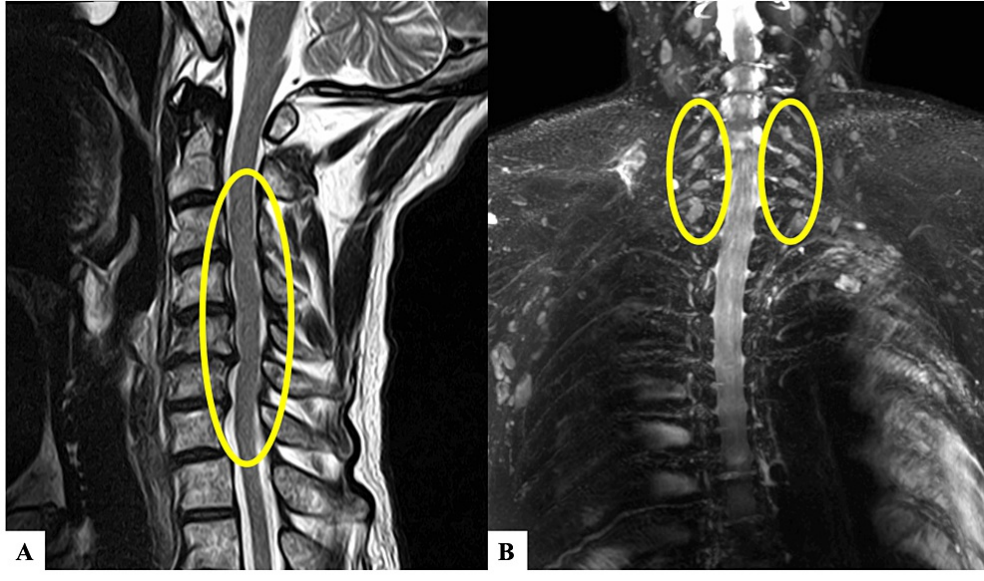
MRI scans (A) Cervical spine plain MRI reveals spinal canal stenosis from C3 to C7, with no changes in signal intensity within the spinal cord (circle). (B) Cervicothoracic spine contrast-enhanced MRI (MR neurography) shows an enlargement of the dorsal root ganglia from C4 to C7 and enhancement with contrast medium (circle).
